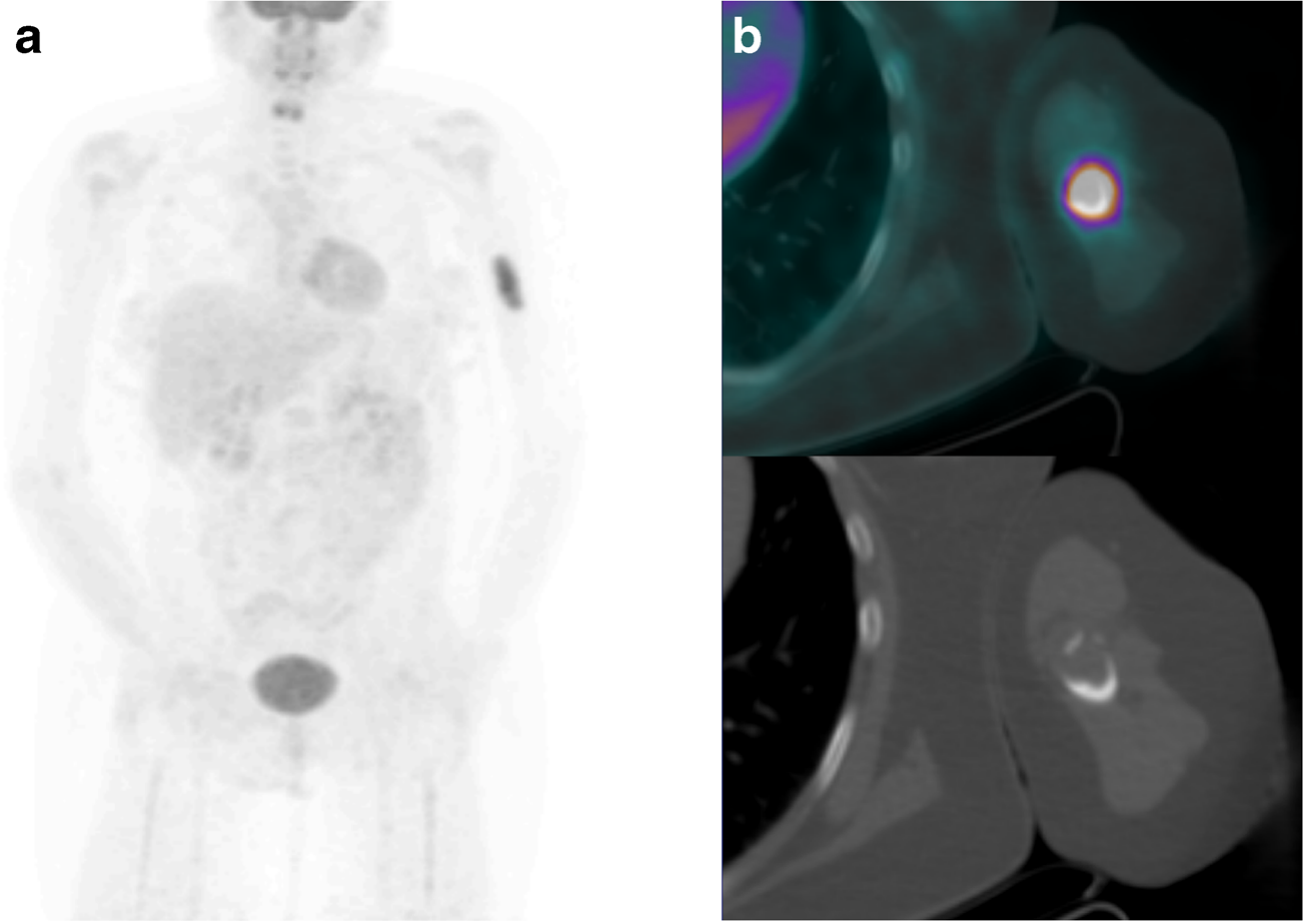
PET-CT imaging performed 3 months post-CRT in a 40-year-old patient with stage 2B disease demonstrates CMR in the pelvis, but a new left humeral bone metastasis (Score 5, PD). This was treated with radiotherapy, but the patient had progressive metastatic disease elsewhere and died 18 months post-initial diagnosis
